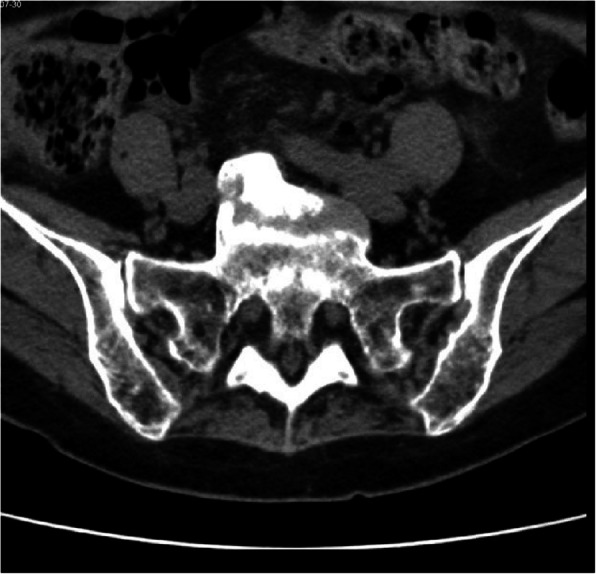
Transverse plane CT scan: sacral promontory
